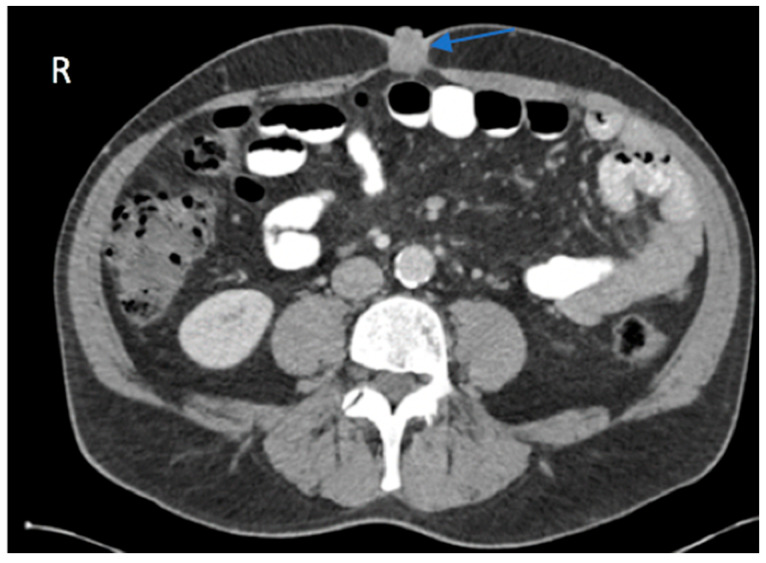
Axial view of the CT of the abdomen and pelvis with contrast showed induration at the umbilicus consistent with the location of the patient’s skin lesion (blue arrow). Biopsy of the lesion was compatible with a Sister Mary Joseph nodule, indicative of the patient’s intrahepatic cholangiocarcinoma.
